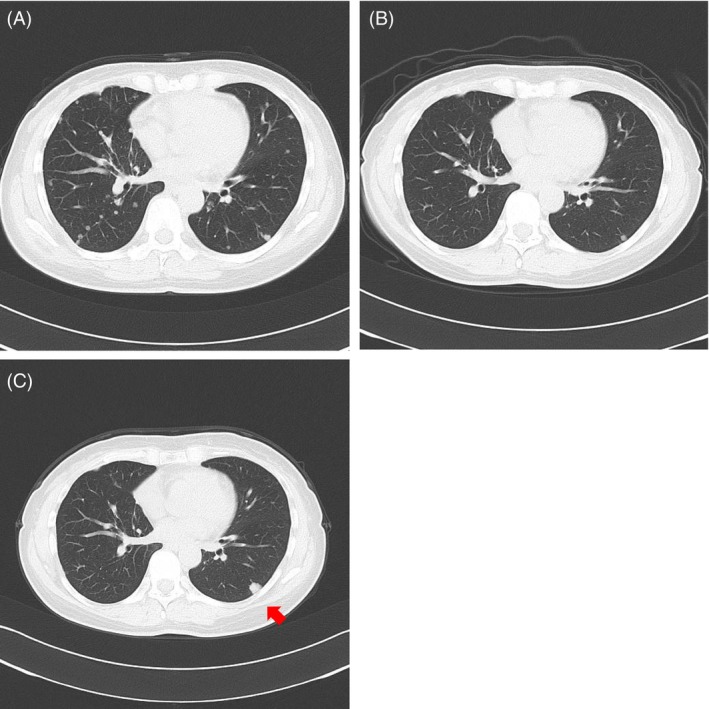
Chest computed tomography obtained (A) 1 year and 9 months, (B) 3 years and 2 months, and (C) 4 years (arrow, enlarged S6 nodule) after lobectomy.
